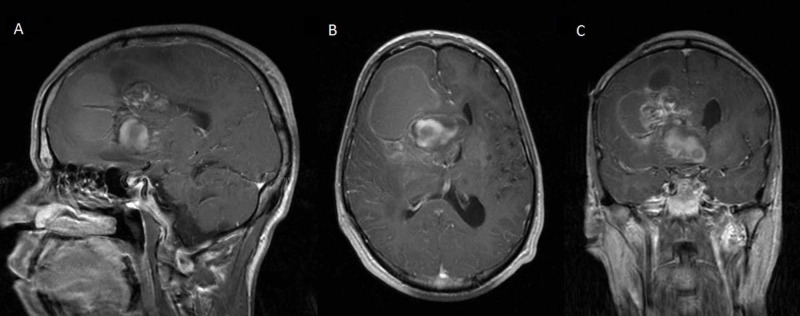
Preoperative sagittal (A), axial (B), and coronal (C) T1-weighted MRI with contrast, showing the solid cystic mass centered near the septum pellucidum. Only faint enhancement after gadolinium injection is seen within the solid mass.
